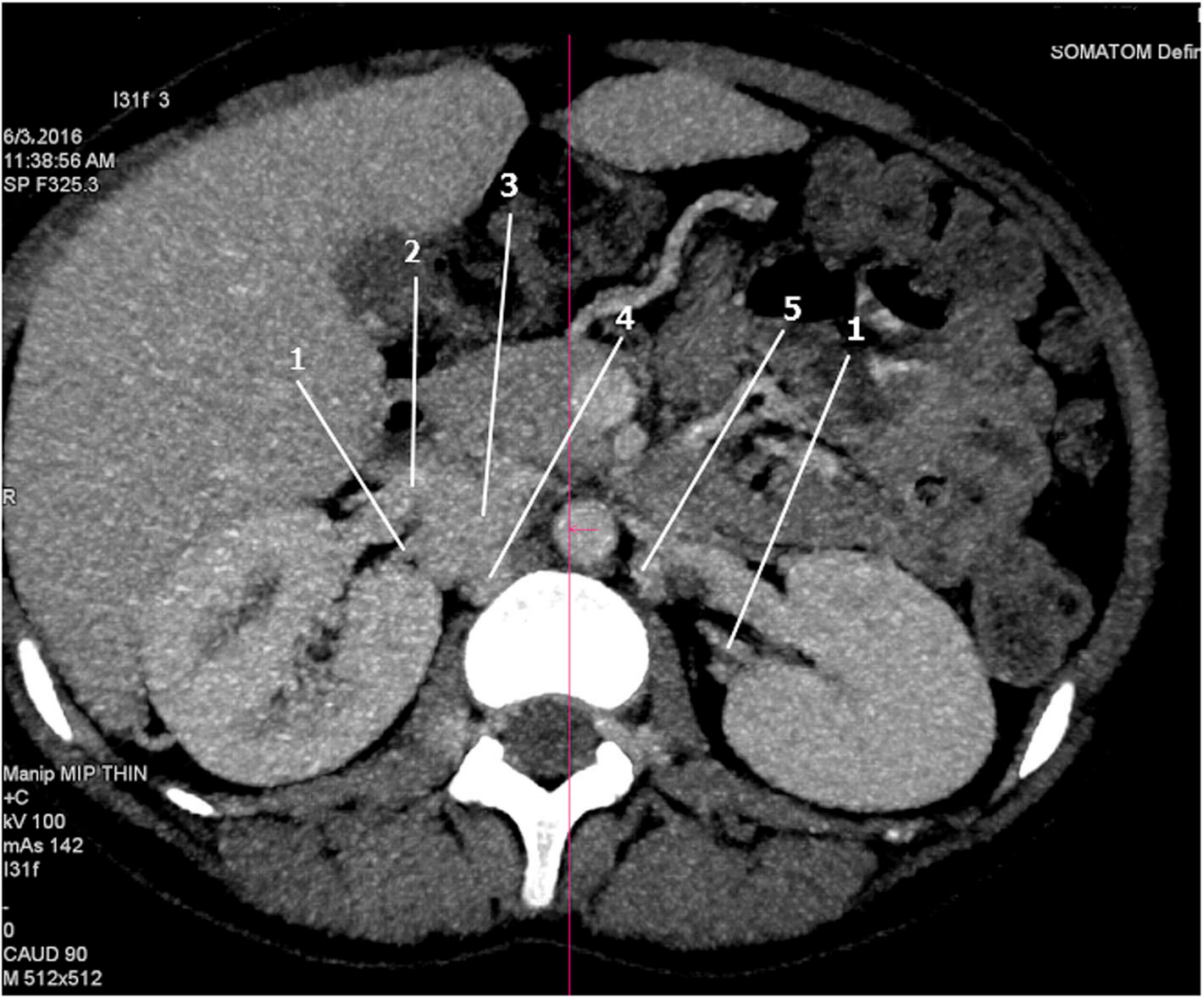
Computed tomography angiography (axial view) of the abdomen displaying posterior divisions of renal veins draining into the hemiazygos and azygos veins respectively. *1* posterior division of renal vein, *2* anterior division of renal vein, *3* inferior vena cava, *4* azygos vein, *5* hemiazygos vein
